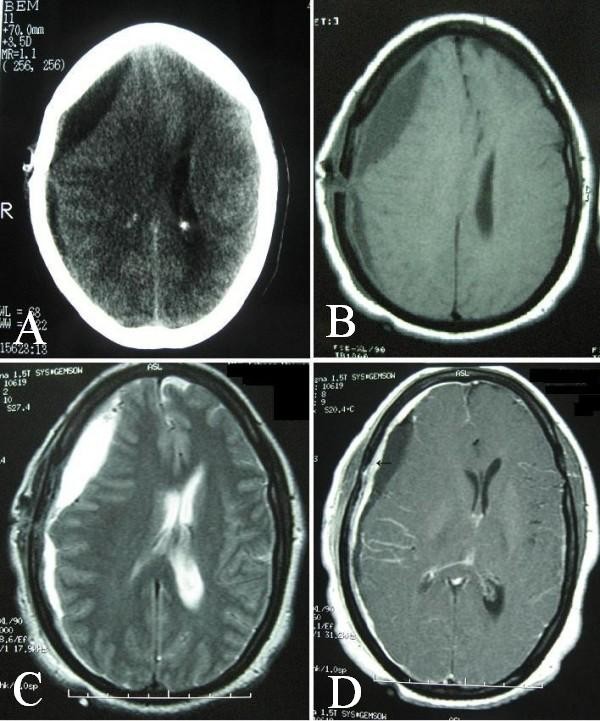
Computed tomography obtained 11 days after surgery showing reaccumulation of the subdural effusion (A). Magnetic resonance images showing right frontoparietal subdural fluid, hypointense in T1-weighted (B) and hyperintense in T2-weighted axial plane (C). Thick dural enhancement (arrow) and a pattern of gyral enhancement at the right parietal region (D).
